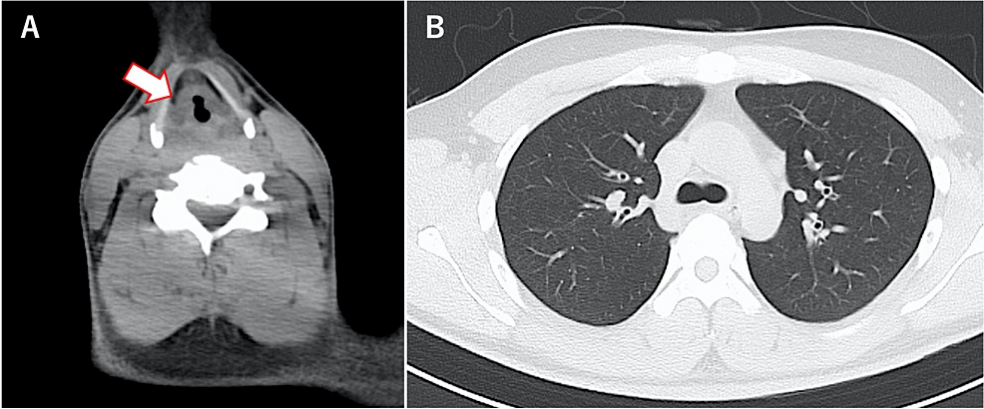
CT image showing mild edema over the vocal cords (A) and normal bronchial walls (B)
